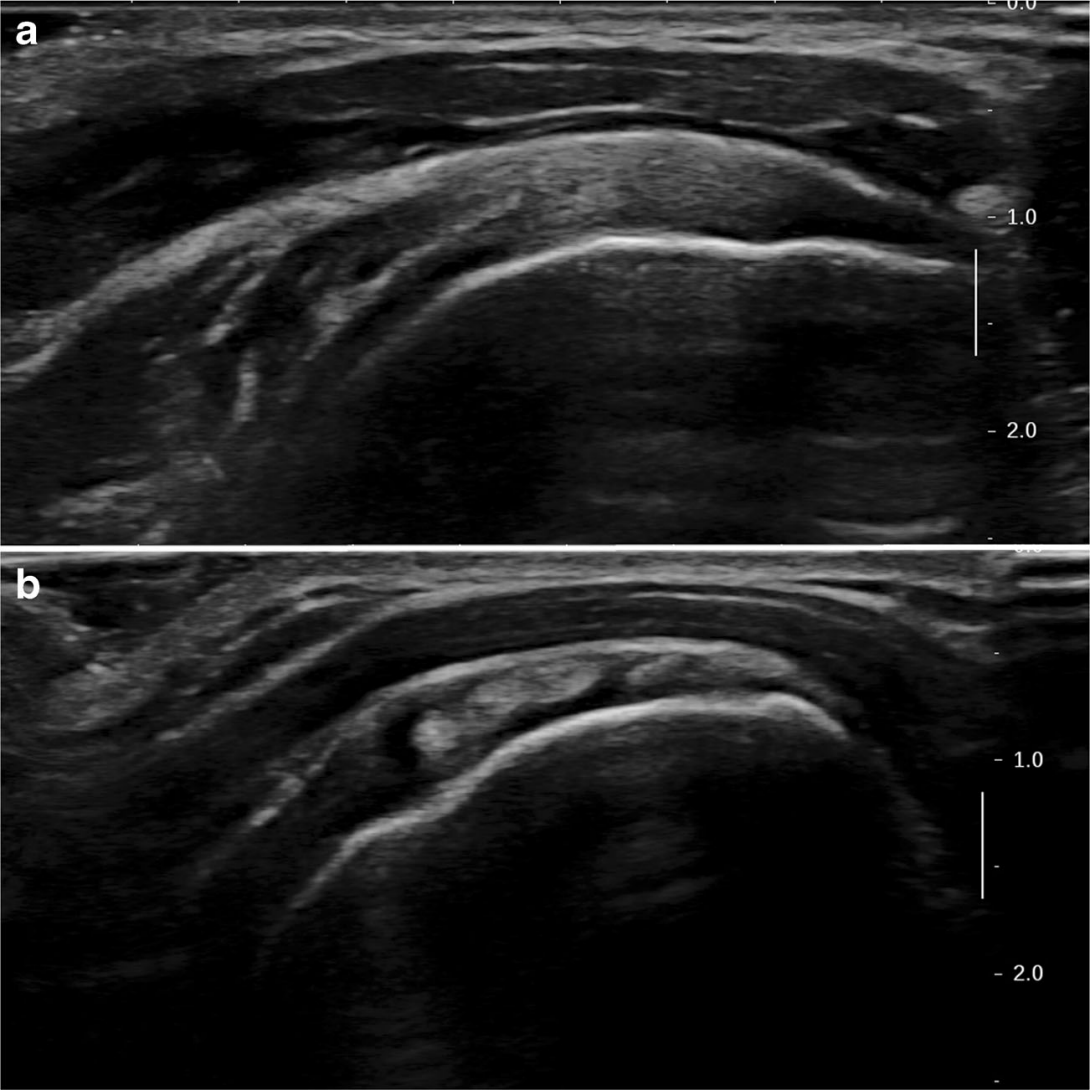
B-mode ultrasound images of the SSC. a Transverse view (long axis) : the normal SSC tendon is hyperechoic compared to the adjacent muscle and has a fibrillary structure. b Sagittal view (short axis): multi-pennate structure of the SSC tendon with alternation of hypo and hyperechoic zones
